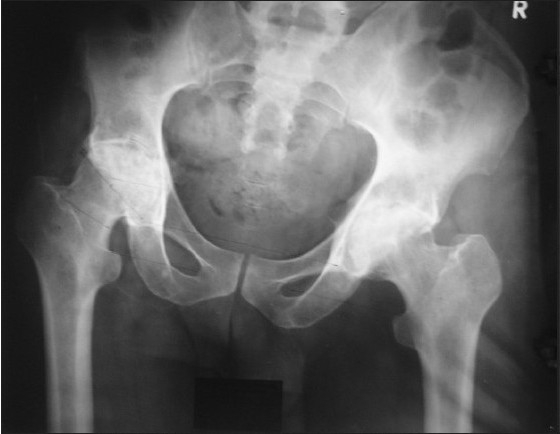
Pre operative X-ray pelvis with both hips showing bilateral femoral head destruction in an inflammatory arthropathy
